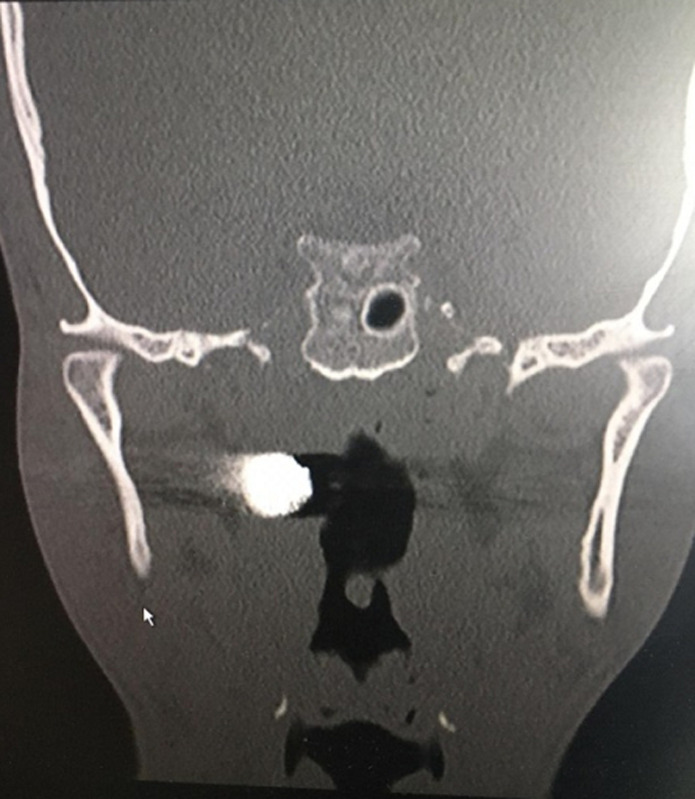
coronal view of the computed tomography scan
